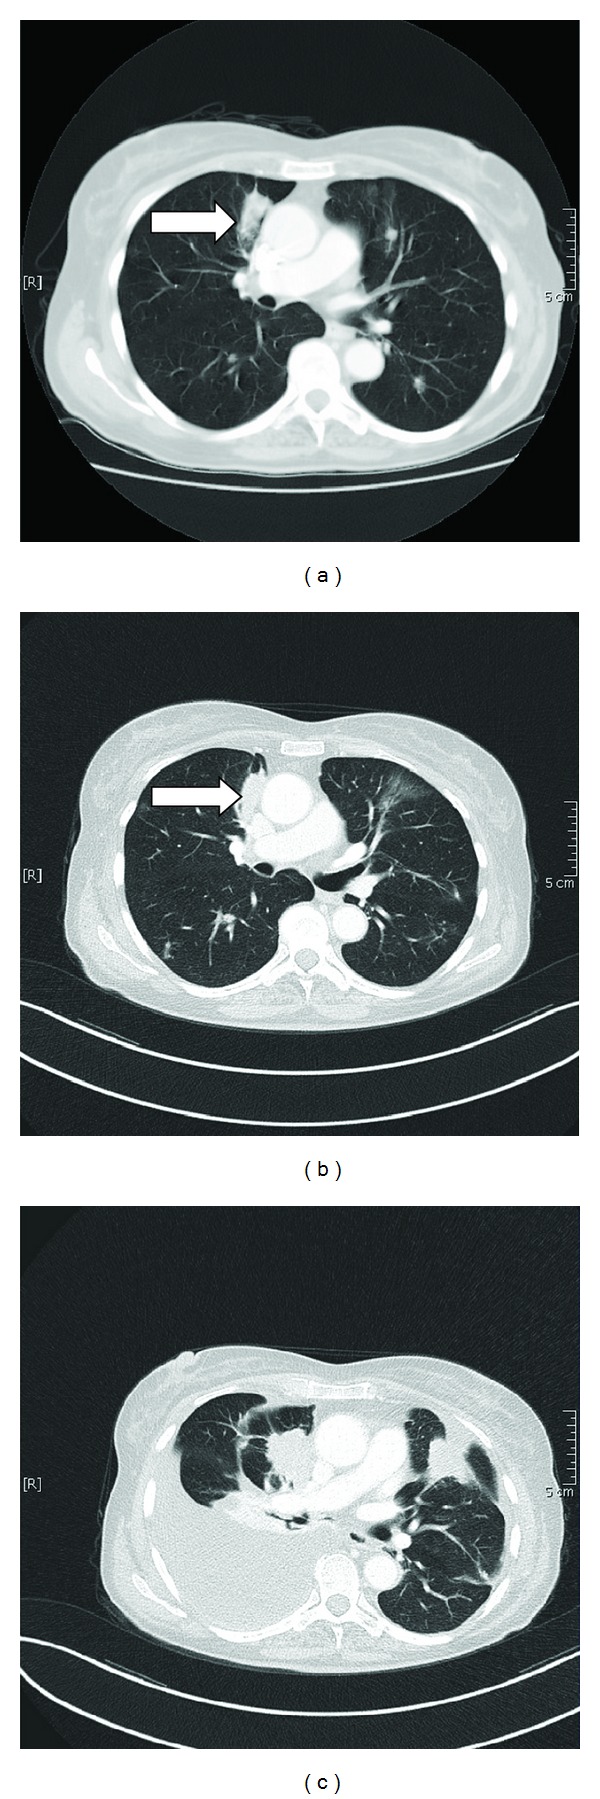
Contrast-enhanced tomography revealed a major pulmonary mass within the right upper lobe (arrow) (a). Stable disease was achieved under first line therapy with carboplatin and pemetrexed (b). CT-evaluation revealed tumor progression of the pulmonary mass and metastases, huge pleural effusion due to pleural carcinosis of the right side (c).
